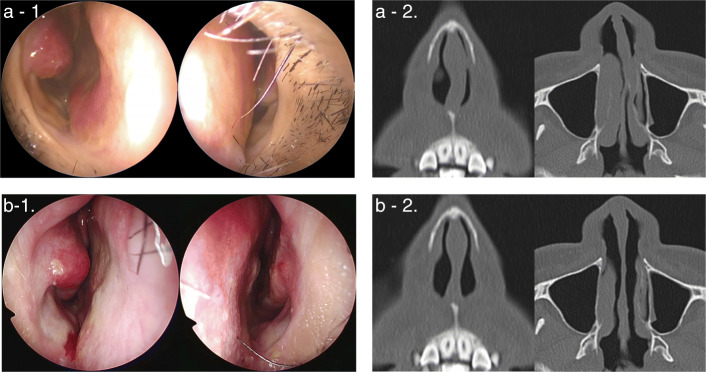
Nasal findings and CT before (a-1, 2) and 3 months after (b-1, 2) the surgery. a-1, 2. Preoperative nasal findings and CT. The left nasal cavity is narrowed by the caudal deviation. b- 1, 2. Postoperative nasal findings and CT. MCAST corrected the caudal deviation and equalised the right and left nasal cavities. CT: computed tomography; MCAST: the modified cutting and suture technique
